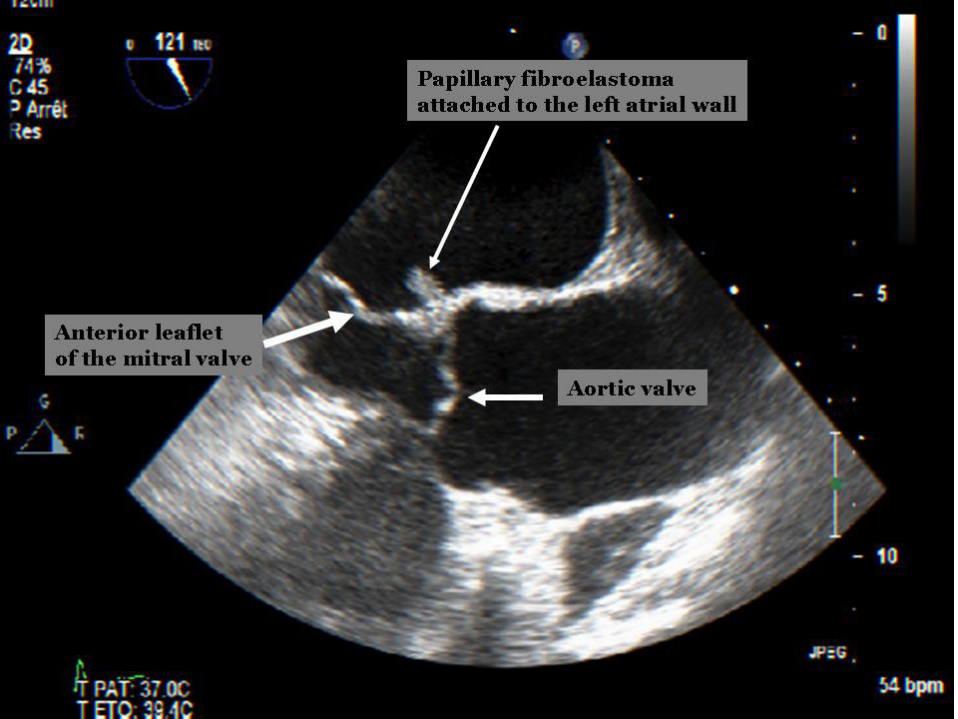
Pre-operative trans-esophageal echocardiographic image showing small mobile mass attached to the left atrial wall on the level of the aortic valve.
